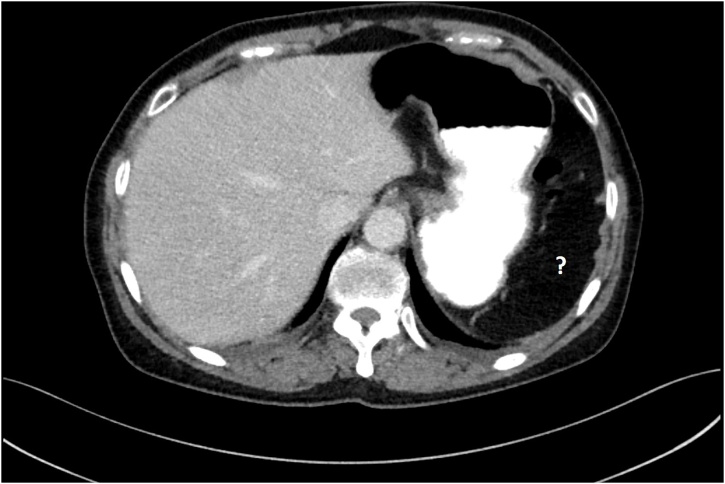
Transverse CT slice showing an absent spleen as denoted by a question mark.
